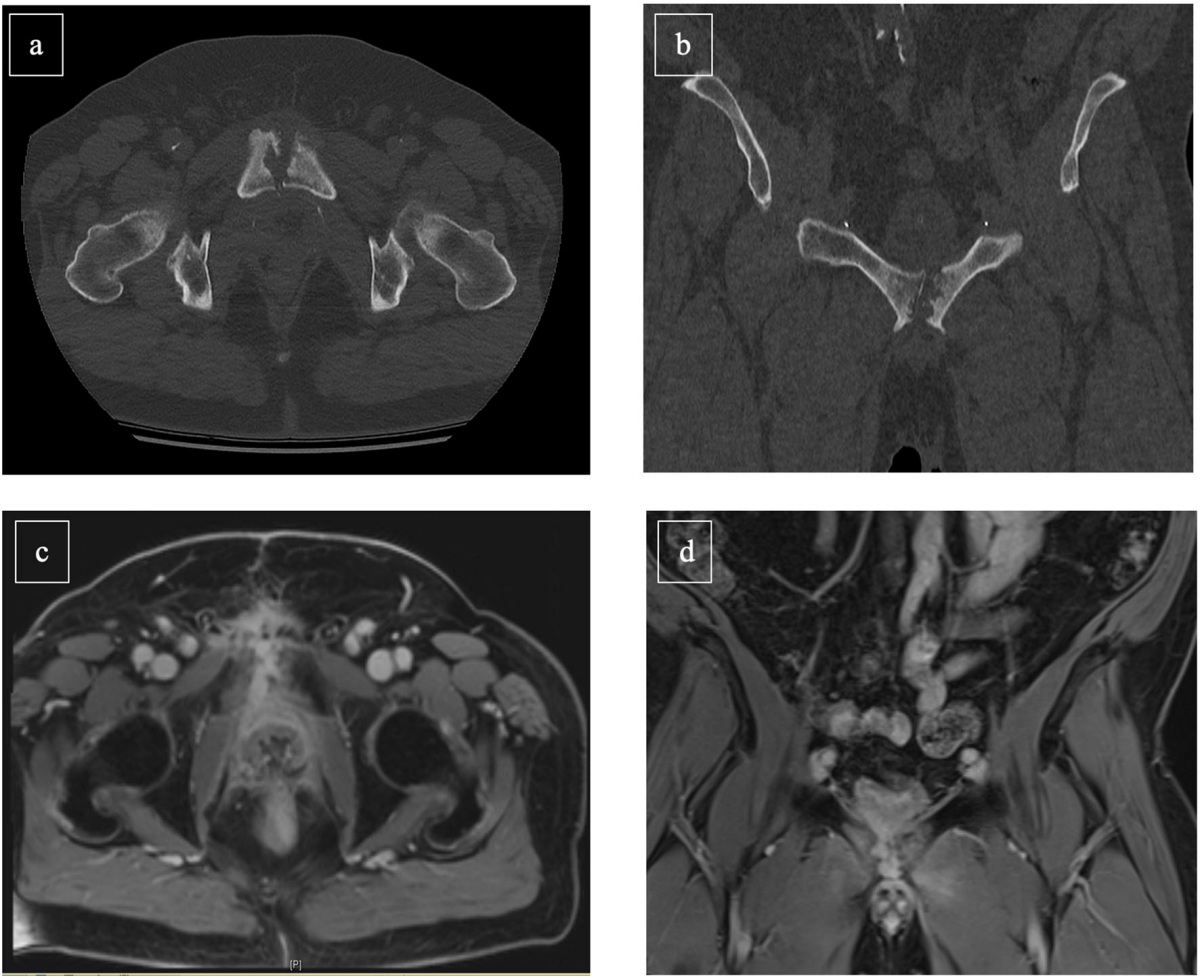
Abdominal CT scan [(a): transversal, (b): frontal] and MR-Imaging [(c): transversal, (d): frontal] of a 56-year-old prostate cancer patient suffering osteomyelitis following photon-beam radiation therapy of the prostate (2017) and salvage radical prostatectomy with persistent insufficient anastomosis (2019). Cystectomy with Mainz-I pouch with appendix-nipple was successful performed. Furthermore, symphyseal resection and omentus major flap was simultaneously achieved.
